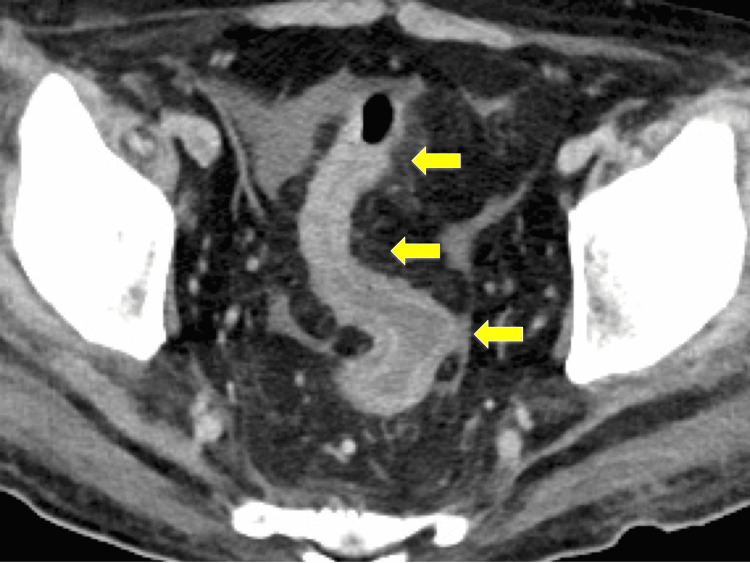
Contrast-enhanced computed tomography. Computed tomography showing edematous changes throughout the colon (arrows).
